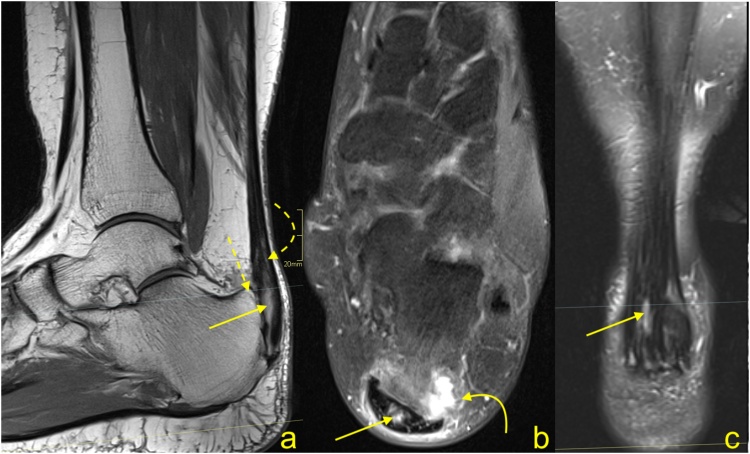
Advanced stage of Haglund's syndrome. A 67-year-old patient with pain at the insertion of the Achilles tendon for more than 5 years. MRI revealed Haglund’s deformity (dashed arrow); alteration in the signal at the insertion, which corresponds to an intrasubstance defect and discrete diffuse calcifications (straight arrow); effusion; and synovitis in the retrocalcaneal bursa (curved arrow). A-PD- weighted, b-PD- weighted with fat suppression, c-T2- weighted with fat suppression.
